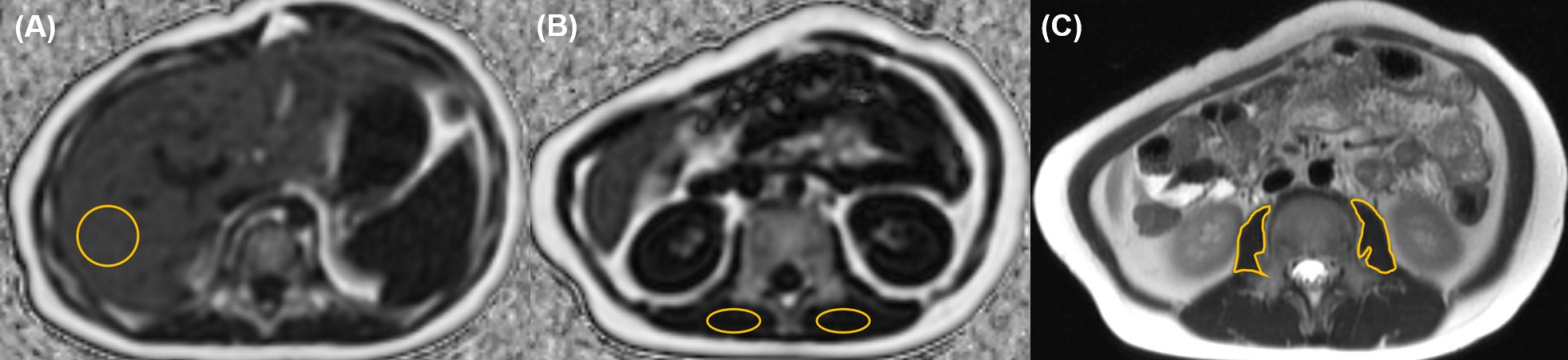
A 7-year-old boy with normal body mass index (18.3 kg/m^2^) and fatty liver. From the liver MRI, (A) fat fraction of the liver was 18.5%. (B) The mean paraspinal muscle fat fraction of the two circles was 2.1%. (C) The PMA was 265.1 mm^2^ and the z-score of PMA was -4.3 in this patient.
